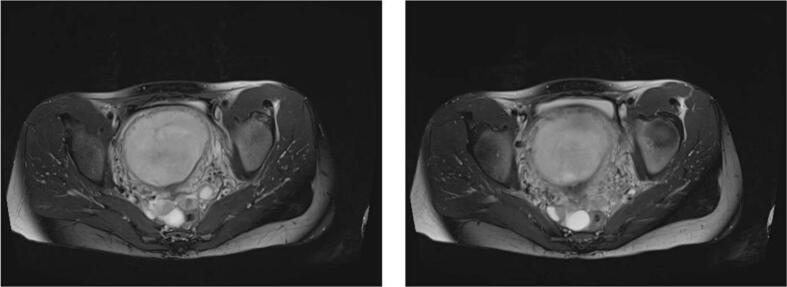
III) July 2018, Small pelvis (Pelvis minor), Axial (transversal sections), Sequence: T2-weighted sequence, No contrast agent administration.
